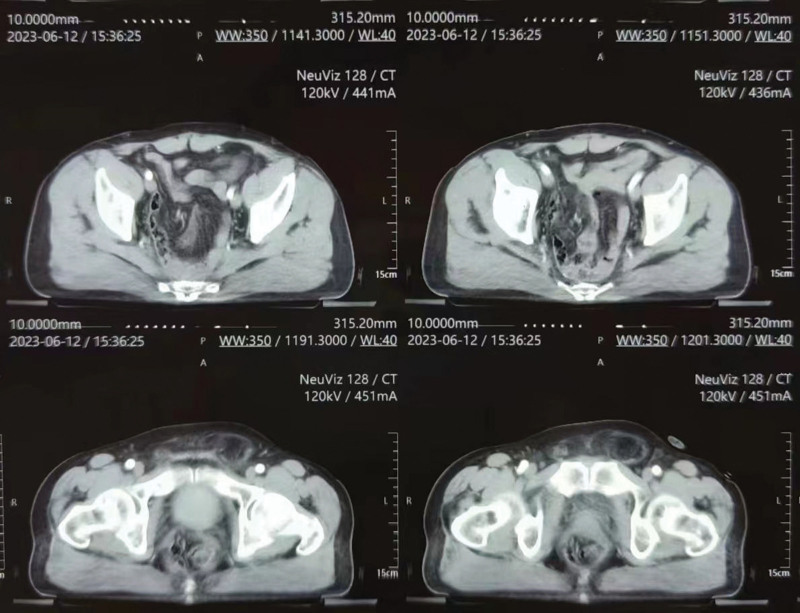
Perineal hernia (PH) was diagnosed on pelvic CT.
